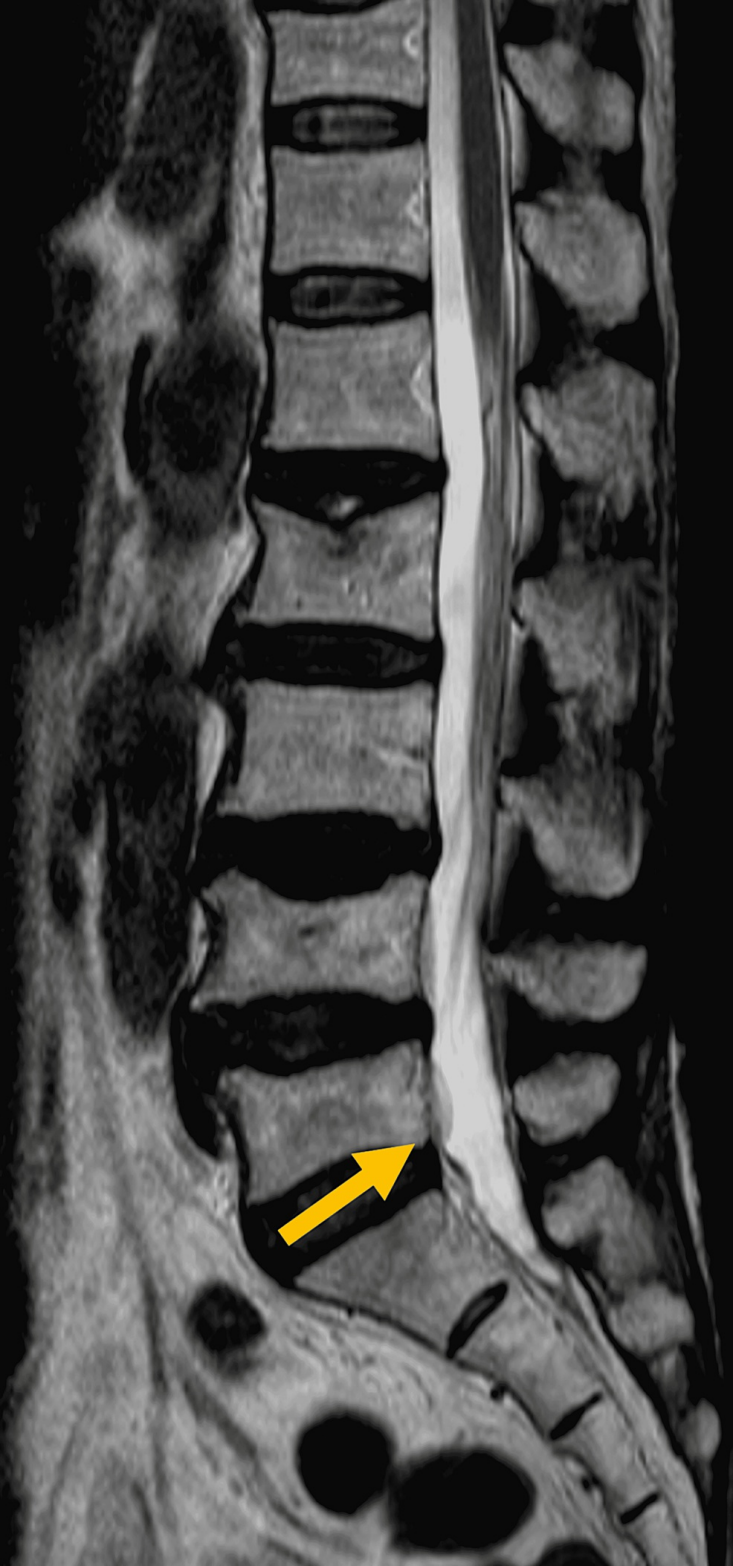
Lumber MRI at one month after onset
